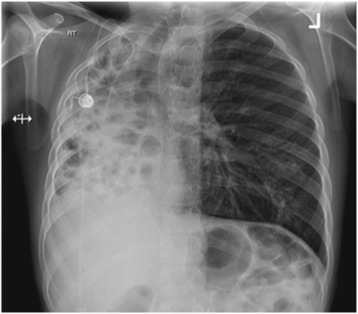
Pre-operative chest radiograph at time of initial assessment showing presence of severe *right* lung collapse and consolidation leading to mediastinal shift to the right with hyperexpansion of the *left* lung
